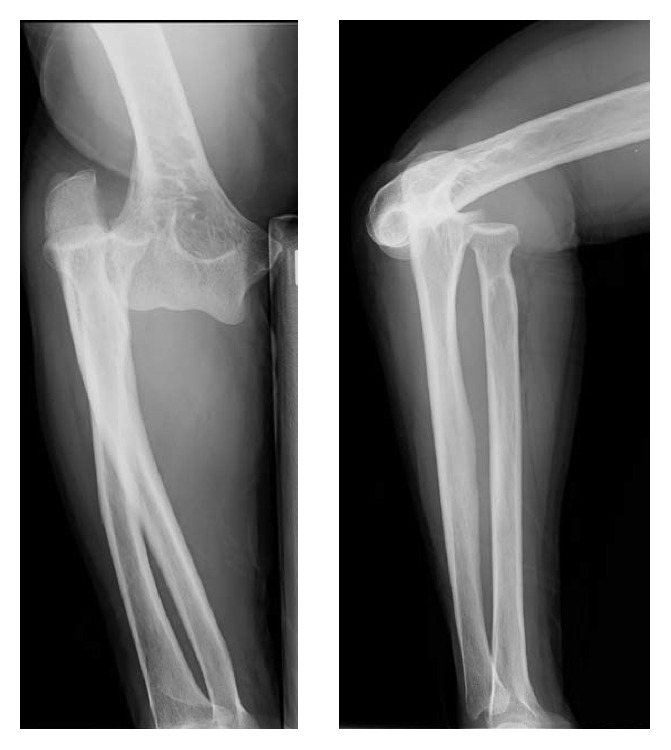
Anteroposterior and lateral radiographs of the elbow revealed lateral convergent displacement of the radius and ulna relative to the humerus without fracture signs.
